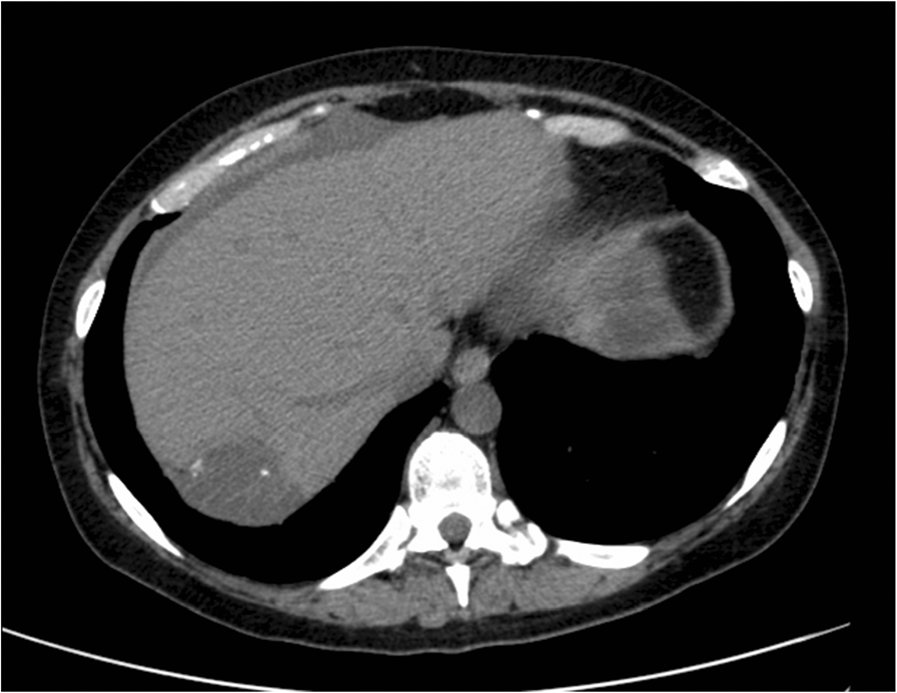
CT scan without contrast of the liver at the time of the first hepatic progression, showing a single metastasis, characterised by pronounced hypodensity and calcification islets (axial plane, abdomen window).
